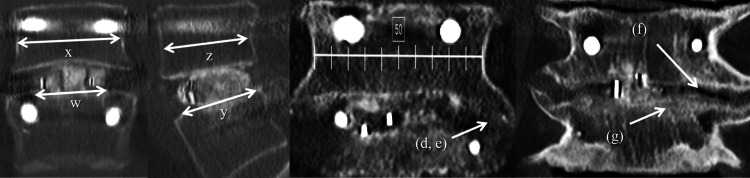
Definition of radiographic parameters using three-dimensional computed tomography scans (a) occupancy rate of autograft in coronal center slice = w/x; (b) occupancy rate of autograft in sagittal center slice = y/z; (c) total occupancy rate of autograft (occupancy rate of autograft in coronal and sagittal center slices) = a×b; (d) osteophyte formation (presence of anterior or lateral osteophytes ≥20% of vertebral diameter); (e) osteophyte union (presence of anterior or lateral osteophyte union); (f) presence of air in the intervertebral disc; (g) presence of osteosclerotic endplate.
